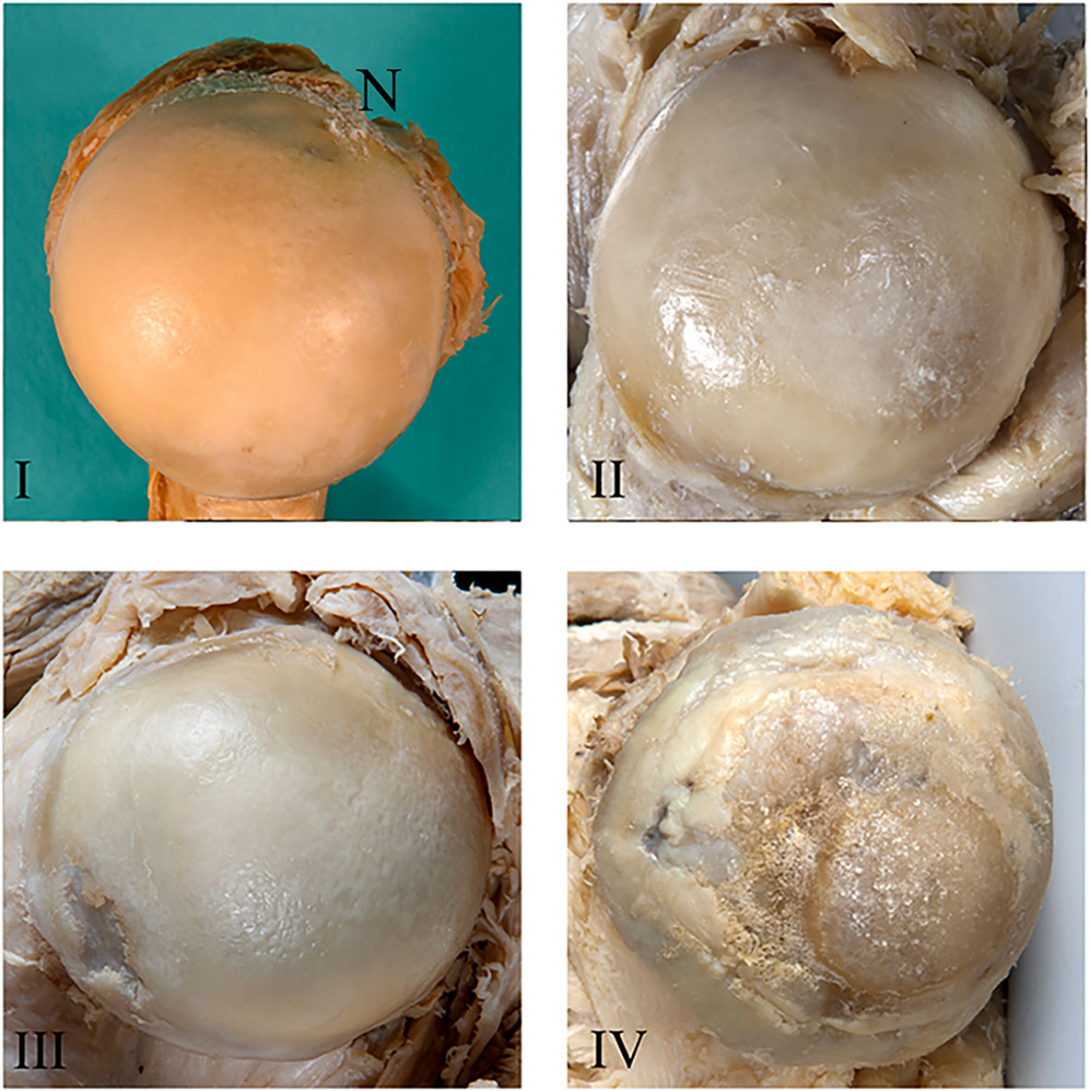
Representative images of osteoarthritis grades I, II, III and IV in dissected humeral heads. (I) N points to the notch in the humeral head formed by the tendon of the long head of the biceps used as a reference for CT-OAM and morphological inspections
